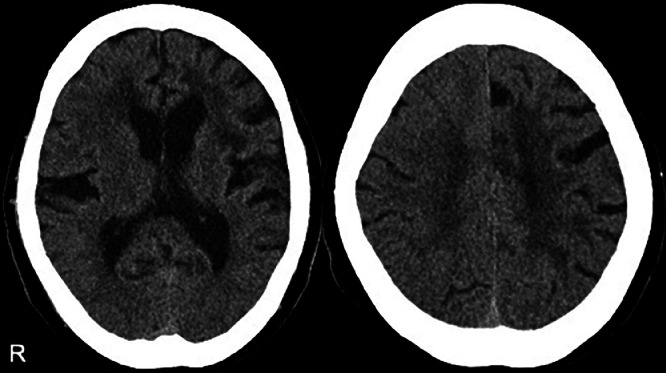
Non-contrast head CT obtained at initial presentation shows no definite acute hemorrhage or mass lesion. No clear established low-density area is identified at this time. This CT was performed as the initial screening examination prior to MRI. The letter ‘R’ indicates the right side of the patient in all images.
